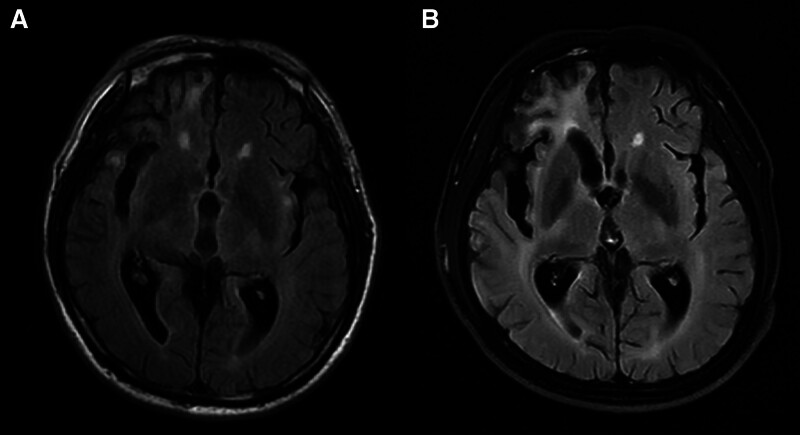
Review of head MRI imaging results after 3 mo of treatment showed a reduction in the patient’s intracranial sheet-like abnormal signal shadow. MRI = magnetic resource imaging.
